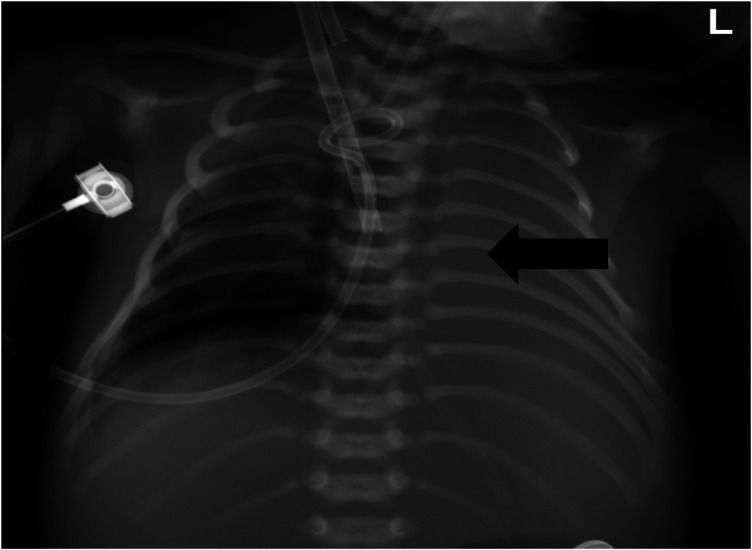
Chest x-ray showed that the right pneumothorax was significantly reduced, and the left lung was not fully expanded.
